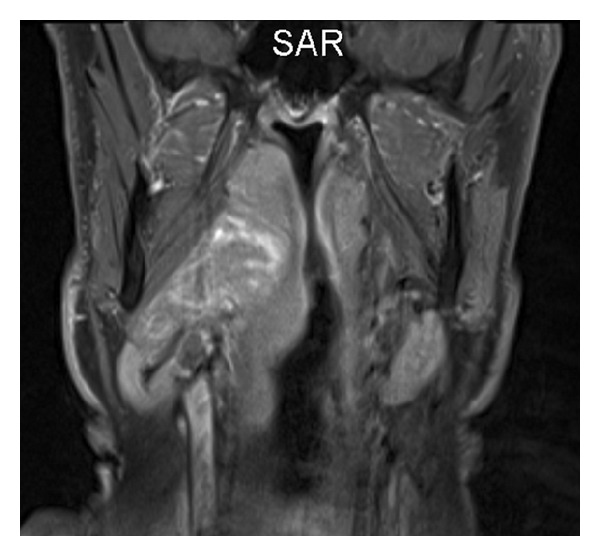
MRI ARM parapharyngeal space with submandibular extension.
